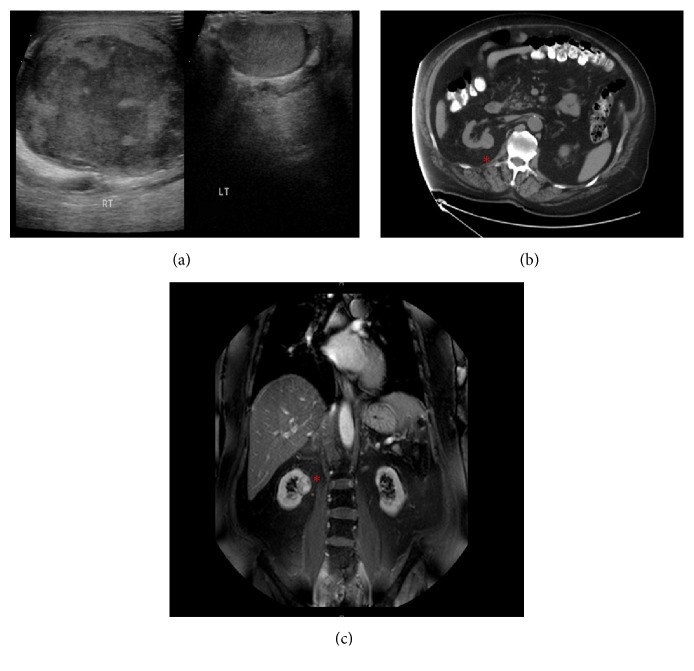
(a) Sonogram of both testes: sagittal view showing grossly enlarged right testis in comparison with the left. (b) T2-weighted MRI of the abdomen with contrast, transverse, and (c) sagittal views; renal cell carcinoma (red asterisk) noted on the right native kidney.
